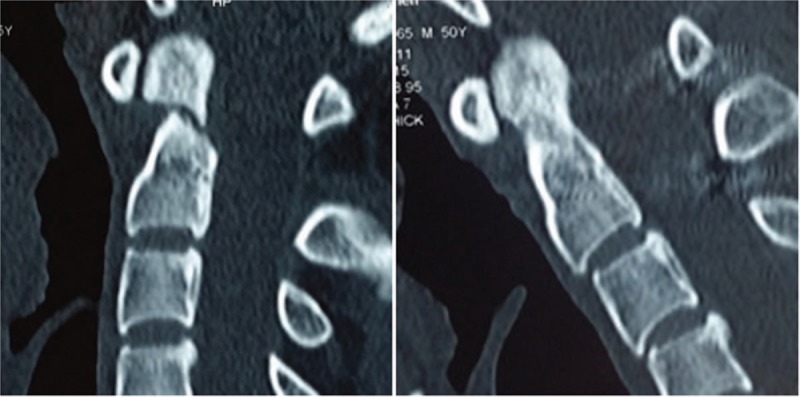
Fracture healing was defined as evident bridging bone across the odontoid fracture site on 1 of the cortices in sagittal CT-scans in neutral head position.
